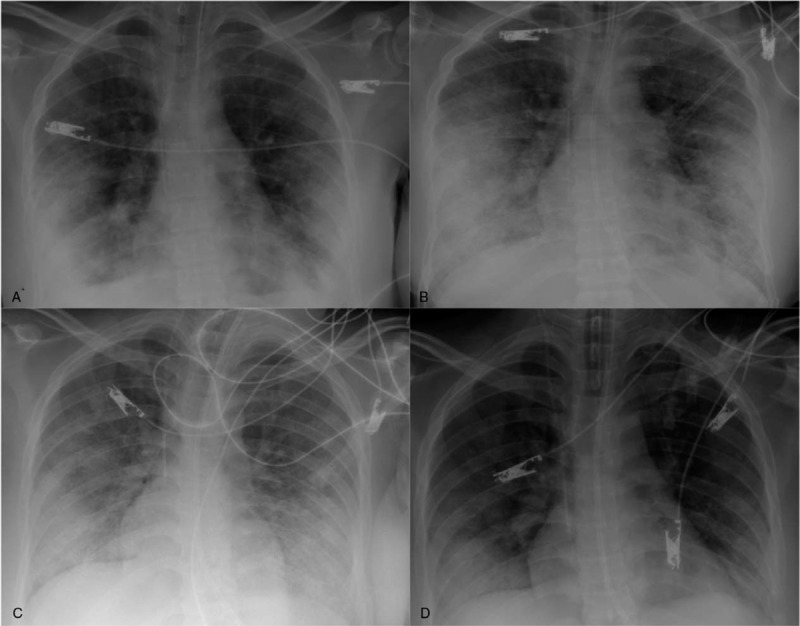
A–D. Chest x-ray evolutionary in time from projection A showing bilateral basal patchy ground glass infiltrates and pleural effusion. B and C. Worsening of the infiltrates occupying the lower two-thirds with greater profusion of the infiltrates of alveolar occupation. D. Chest x-ray with decreasing infiltrates remaining in the bases, which corresponds to the final days of mechanical ventilation.
